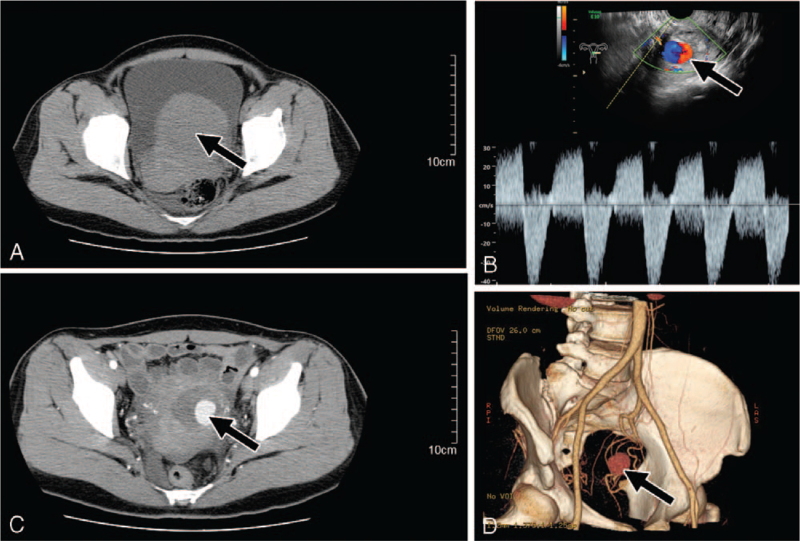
A uterine artery pseudoaneurysm developed after laparoscopic-assisted myomectomy in a 47-year-old patient. (A) Computed tomography showing 7.0 cm subserosalmyoma in the lower left anterior uterine wall (arrow); (B) Color Doppler ultrasonographic image showing swirling blood flow in the myomectomy scar (arrow) on postoperative day 19; (C) Magnetic resonance imaging revealing the enhanced pseudoaneurysmal sac-like structure within the uterus (arrow); (D) Three-dimensional computed tomography angiography showing the pseudoaneurysm originating from the left uterine artery (arrow).
